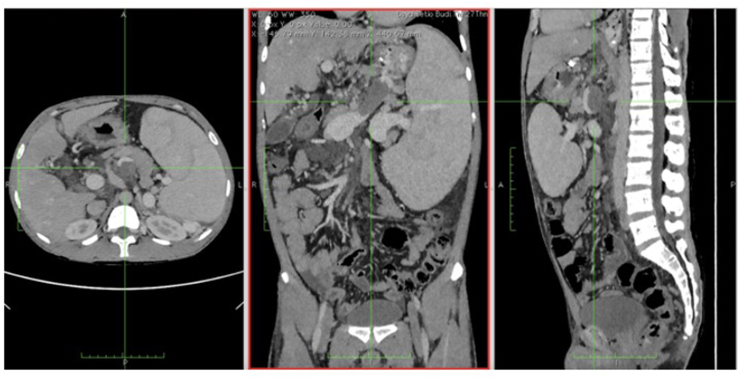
A. Abdominal ultrasound showing liver cirrhosis with hypertension portal; B: serial esofagogastroduodenoscopy (EGD) on day 14, day 17, and one month after hospitalization; C: Abdominal CT with contrast showed thrombus in splenic vein along with splenomegaly.
